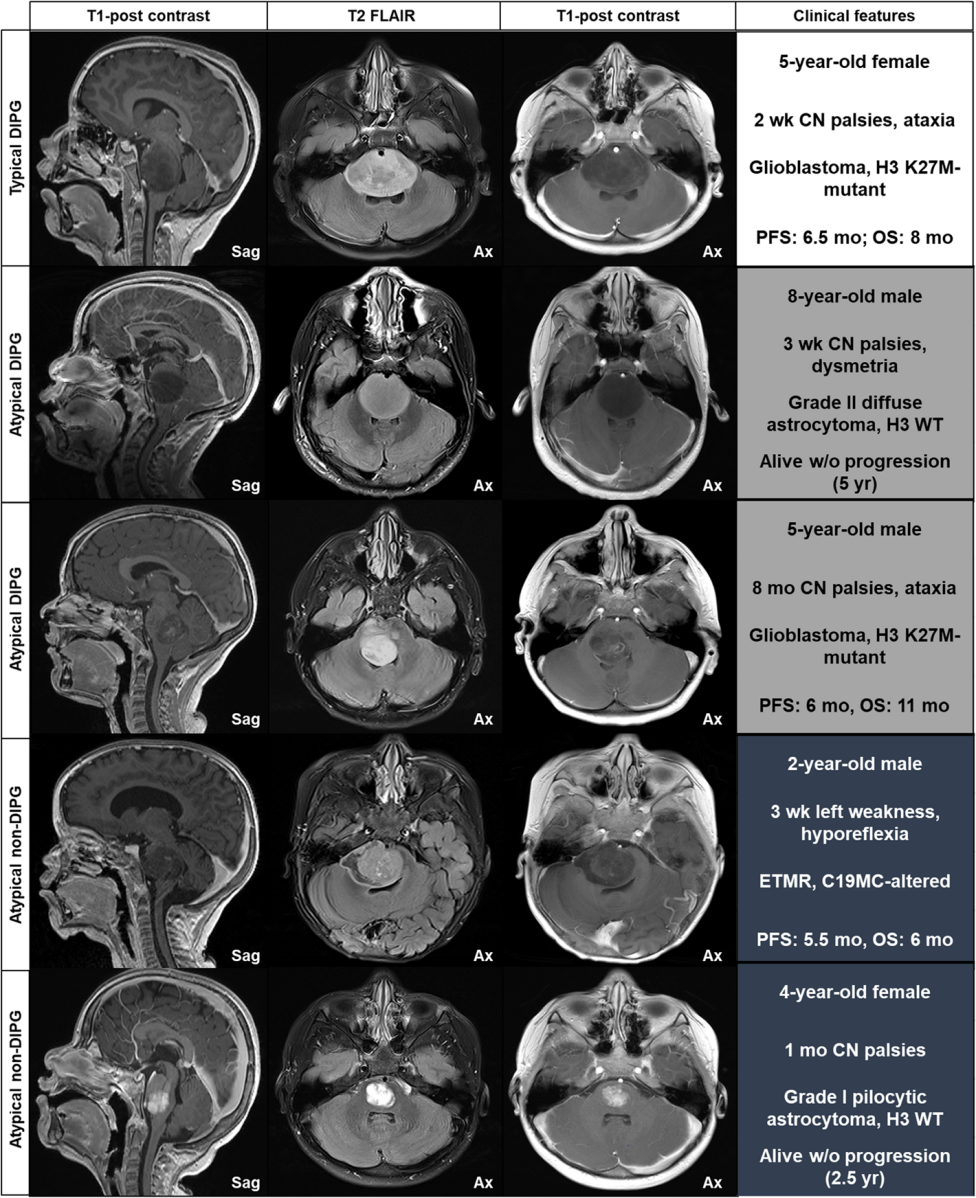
Imaging features of DIPG. The DIPG type is indicated at the left of each row, and the MRI sequence is indicated in the column headers. Clinical features, including patient age and sex; type and duration of neurologic symptoms; pathology; and outcome, with follow-up time indicated in parenthesis for living patients, are shown in the right column. Atypical imaging features included: 2nd row, lack of intralesional inhomogeneity and well-defined margins; 3rd row, tegmental epicenter with dorsal exophytism; 4th row, well-defined margins with diffusion restriction (not shown); 5th row, small focal tumor with uniform avid enhancement. Sag, sagittal; Ax, axial; CN, cranial nerve; PFS, progression-free survival; OS, overall survival; ETMR, embryonal tumor with multilayered rosettes; w/o, without
